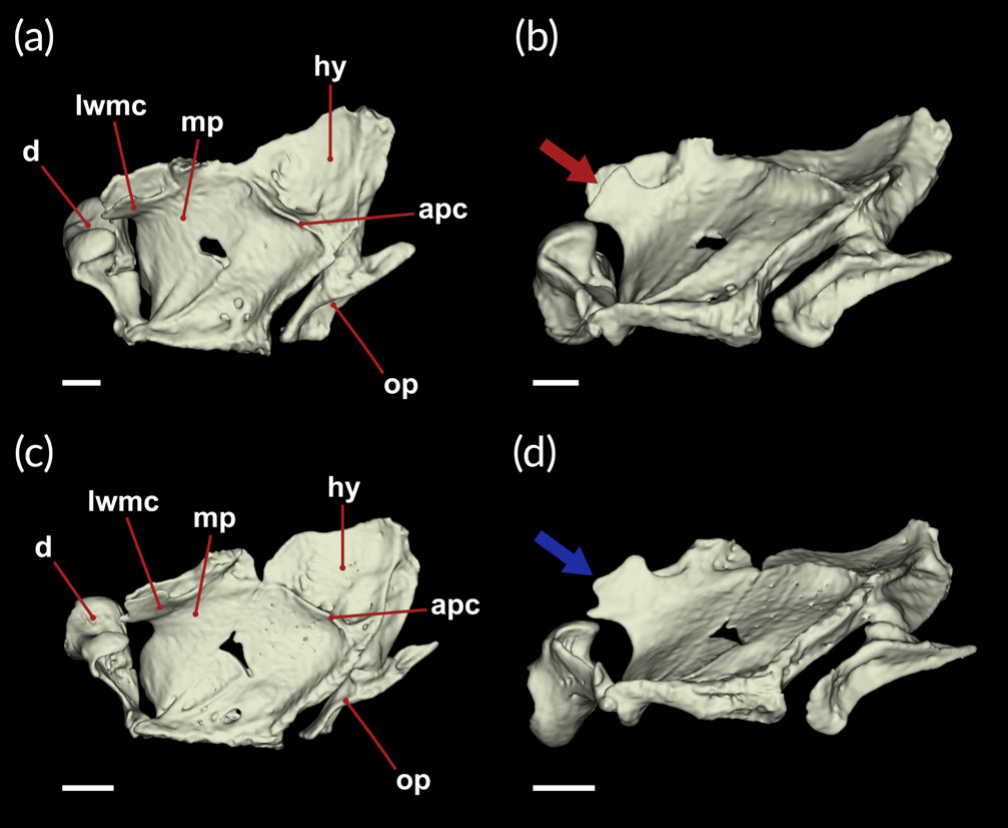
Three‐dimensional computed tomography renderings of the suspensorium in dorsal view. (a) Holotype of *Peckoltia amjikin* (MPEG 40735); (b) lateral view of the suspensorium highlighting the lateral wall of the metapterygoid channel with sinuous edges; (c) suspensorium *of Peckoltia vittata* (MPEG 13428) in dorsal view; (d) lateral view of the suspensorium of *P. vittata* highlighting the lateral wall of the metapterygoid channel and its ornamentation. apc, adductor palatini crest; d, dentary plate; hy, hyomandibula; lwmc, lateral wall of metapterygoid channel; mp, metapterygoid; op, opercle. Scale bar = 1 mm.
